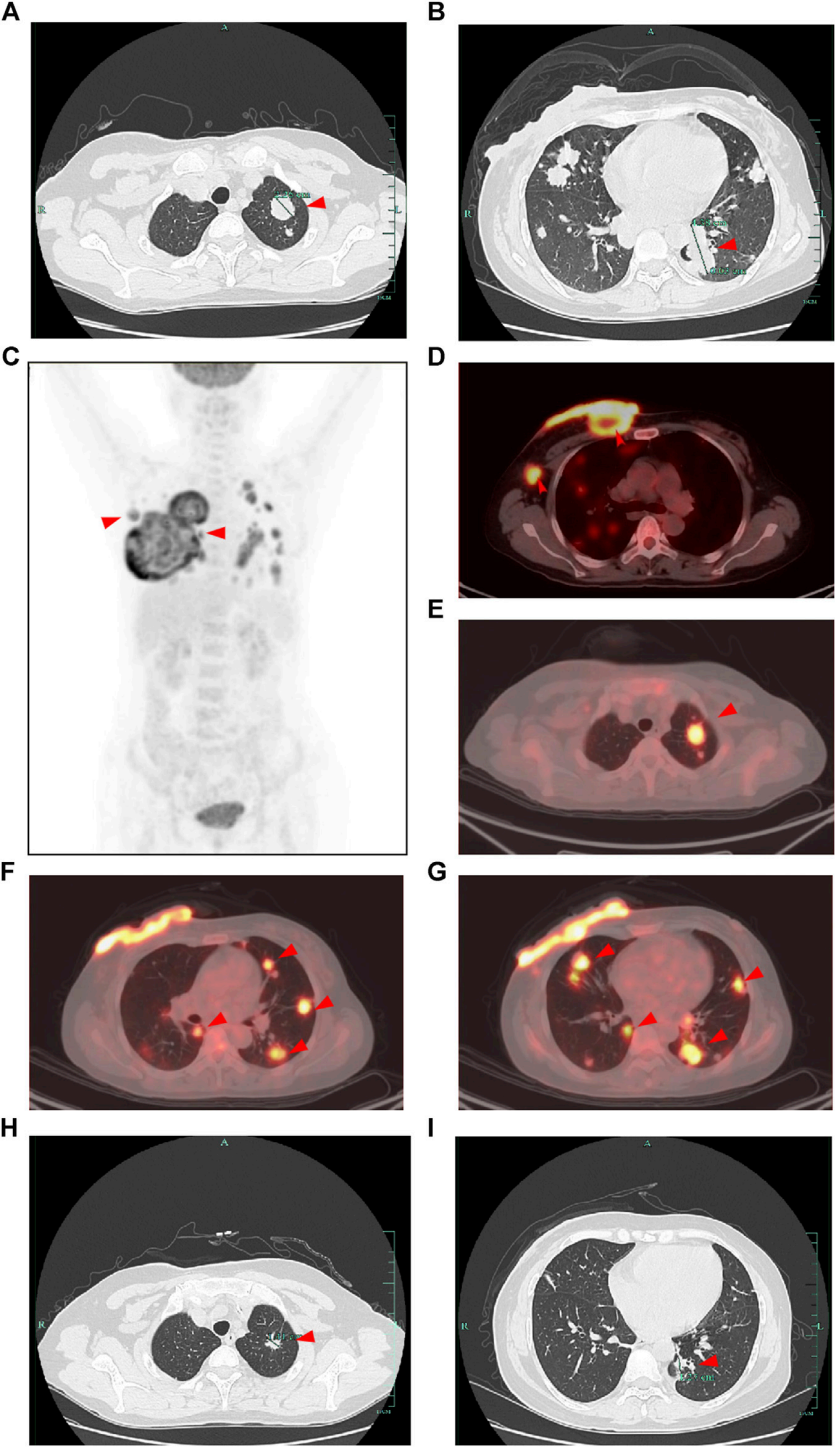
CT and PET-CT images of the patient. (A,B) CT images of the lung at the initial diagnosis showed multiple lung metastasis (shown by the red arrow). (C) Overview of the whole-body PET-CT image. (D–G) PET-CT image showed an increased uptake of fluorodeoxyglucose (FDG) in right chest wall soft tissue, right axillary lymph nodes, and multiple parts of both lungs (shown by the red arrow). (H,I) CT images of the lung after 2 cycles of treatment showed decreased lung metastasis (shown by the red arrow compared with initial diagnosis).
